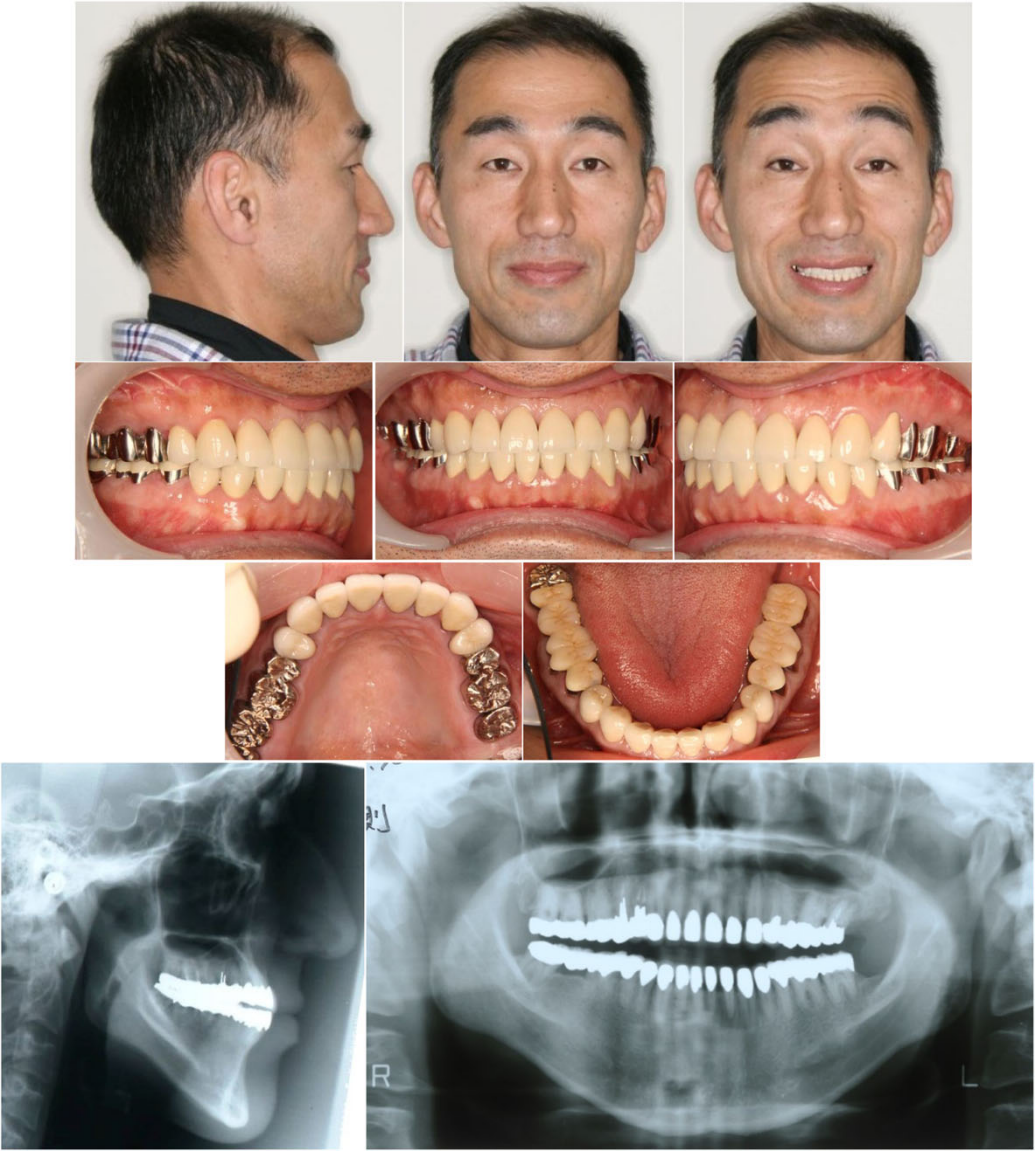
Facial and intraoral photographs, lateral cephalogram and panoramic radiograph at eight-year postretention
